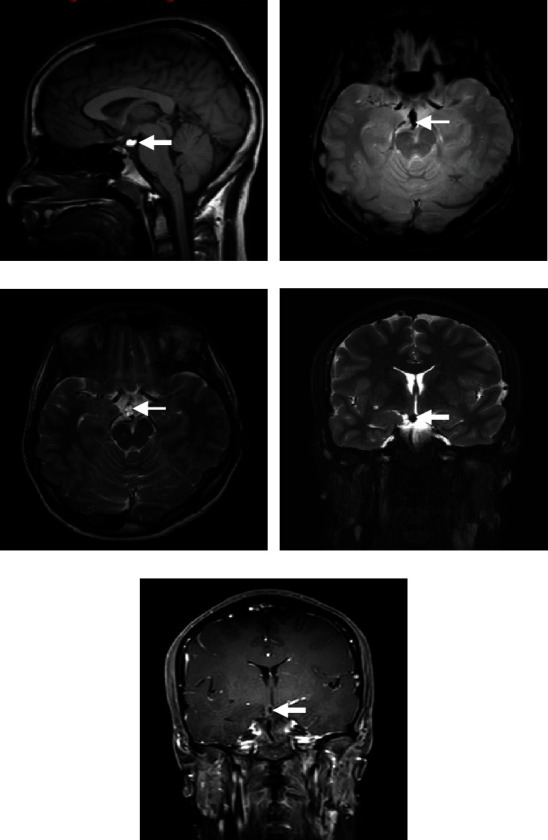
(a) Sagittal T1-weighted MRI. (b) Axial T2-gradient MRI. (c) Axial T1-weighted MRI. (d) Coronal T2-weighted MRI. (e) Coronal T1-weighted MRI with contrast. (a)–(e) The images are demonstrating a well-defined hypothalamic lesion following fat signal on all sequences. Faint peripheral enhancement is noted after contrast administration.
